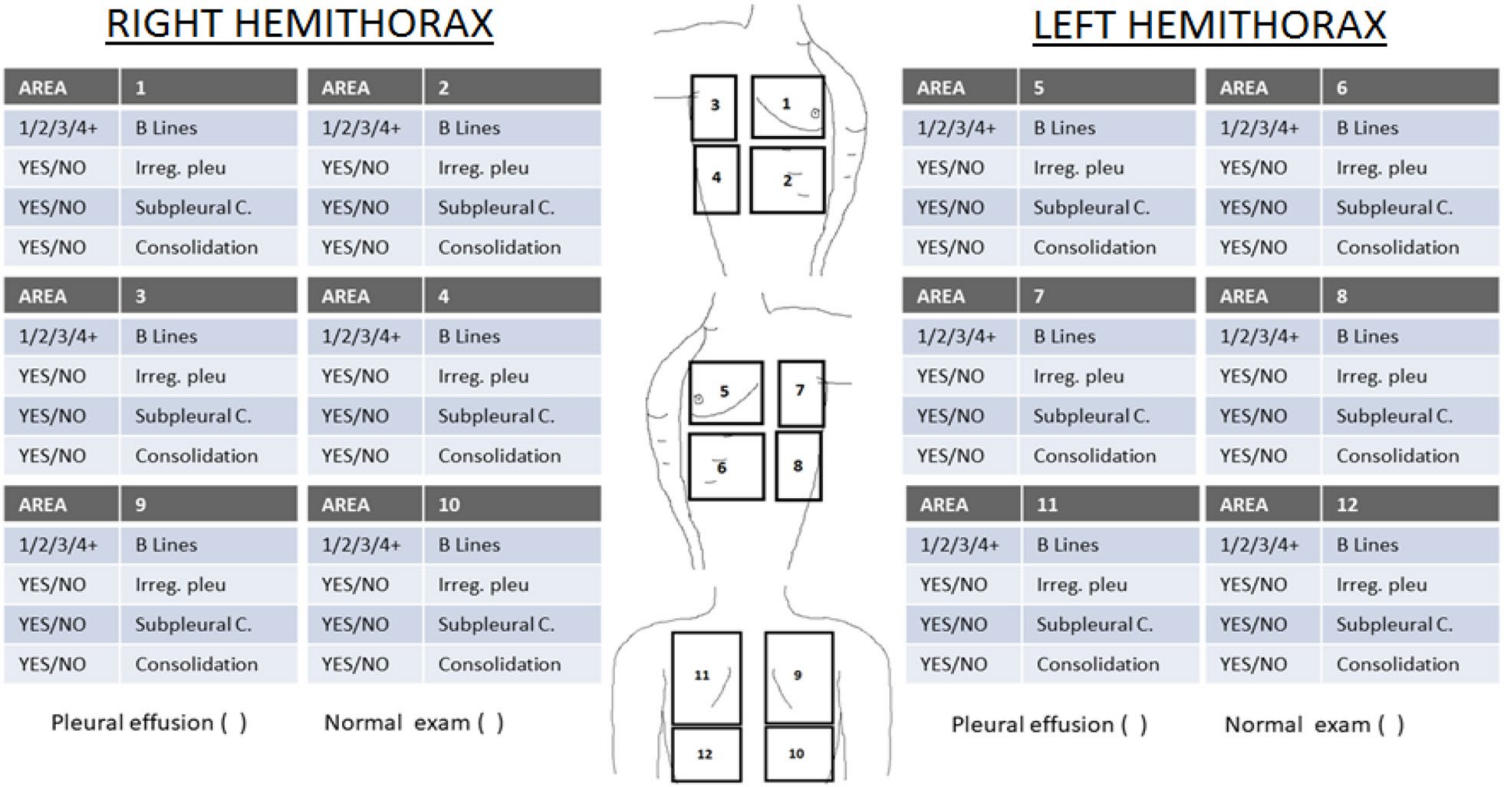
Table for ultrasound data collection. *Irreg. pleu* irregular pleural line, *Subpleural C* subpleural consolidation
